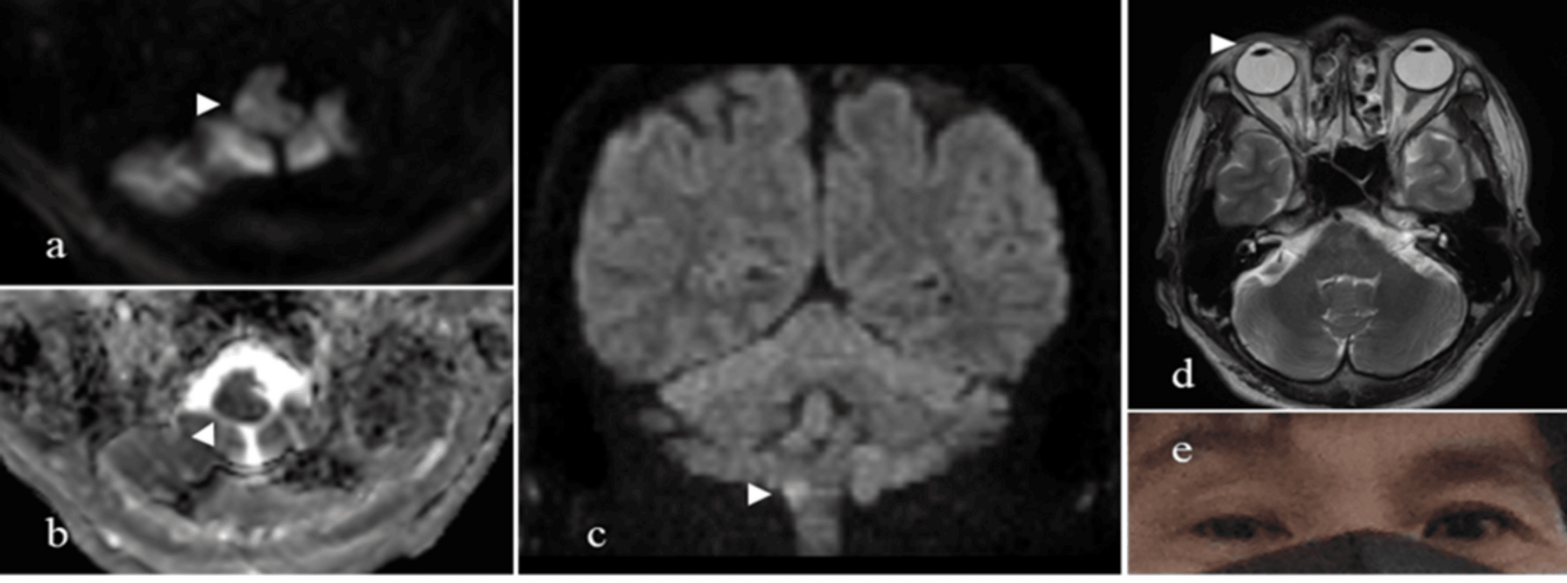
Magnetic resonance imaging findings of the patient (a) Diffusion-weighted imaging (DWI) showing high signal intensity in the right dorsolateral medulla indicating an acute infarction. (b) Apparent diffusion coefficient (ADC) map demonstrating corresponding low signal intensity. (c) Additional DWI confirming the area of infarction. (d) Ocular tilt reaction (OTR) observed in the right eye. (e) Deviation of eye position indicating vestibular imbalance. Arrowheads indicate the site of infarction and gaze deviation.
